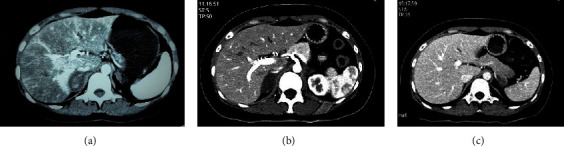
Enhanced bilateral adrenal CT: (a) bilateral adrenal masses with a significantly strengthened signal at the center of both the glands at onset; CT scan values on the left and right adrenal glands were 30 HU and 58 HU, respectively. (b) Two low-density nodular shadows located on both adrenals 4 days later. CT scan values on the left and right adrenal glands were 15 HU and 12 HU, respectively. (c) Atrophy of adrenal glands in the third-month follow-up. CT scan values on the left and right adrenal glands were 30 HU and 38 HU, respectively.
